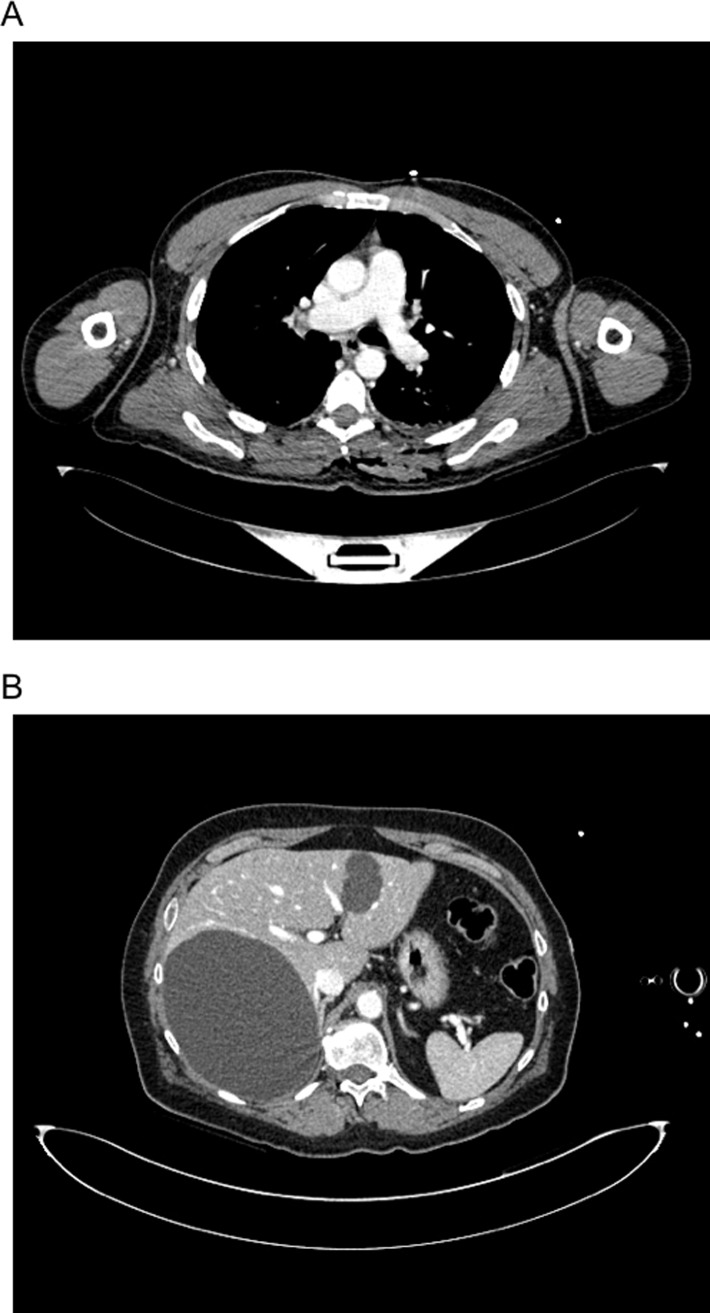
Feasibility of contrast enhanced CT via i.o. access with very good enhancement of the major thoracic vessels (A) and parenchymatous upper abdominal organs (B).
